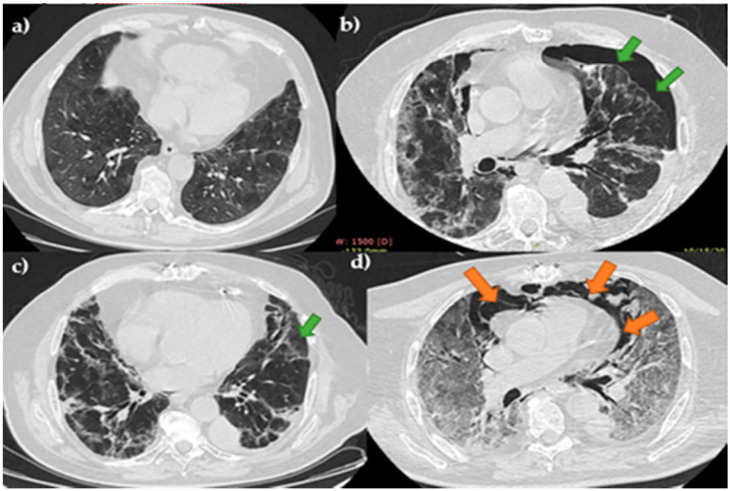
Case 1–patient admitted with moderate COVID-19 injury, developed PT and subsequently PM. Legend: PT-pneumothorax—green arrow; PM-pneumomediastinum—orange arrow; (a) initial CT with moderate injury; (b) right PT; (c) control CT after surgical drainage of PT; (d) PM and severe pulmonary injury.
